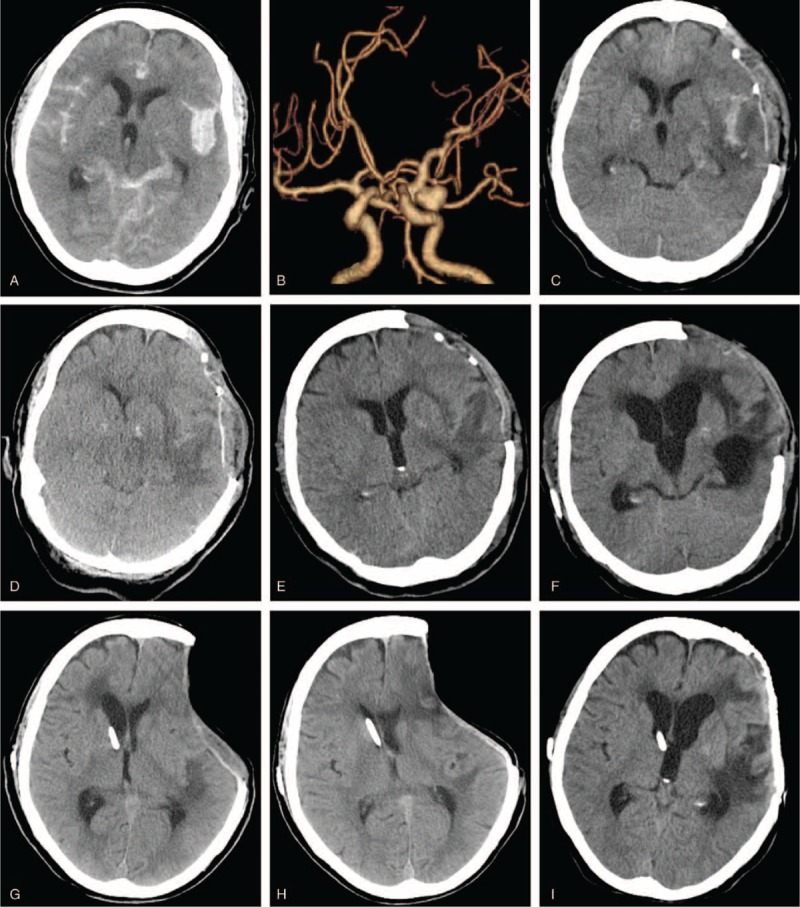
Imaging findings in the case with 2 episode of paradoxical herniation. A, CT scan showing subarachnoid hemorrhage (SAH), a hematoma in the left sylvian fissure, brain swelling, and no midline shift upon admission. B, CTA scan revealing an aneurysm at the terminal segment of the carotid artery. The patient underwent emergency decompressive craniectomy, followed by epidural drain. ICP returned to normal range. The patient developed high fever. On postoperative day (POD) 6, a lumbar puncture was performed, and provided evidence to rule out pyogenic meningitis. D, CT scan on POD 16, showing paradoxical herniation. At this time, the GCS score was 5; BP was 81/38 mm Hg; ICP was 2 cm H_2_O. The patient responded to rapid hydration and Trendelenburg position. E, CT scan on POD 23, showing resolution of the midline shift. On POD 36, neurological function deteriorated. F, CT scan showing enlarged ventricular system with external brain tamponade. The patient received emergency ventriculoperitoneal shunt. G, CT scan on POD 44 showing much smaller ventricular system. H, CT scan on POD 62, showing the second episode of paradoxical herniation. I, Cranioplasty on POD 65. The patient responded to treatment, and recovered to discharge. CT = computerized tomography, GCS = Glasgow Coma Scale, ICP = intracranial pressure.
